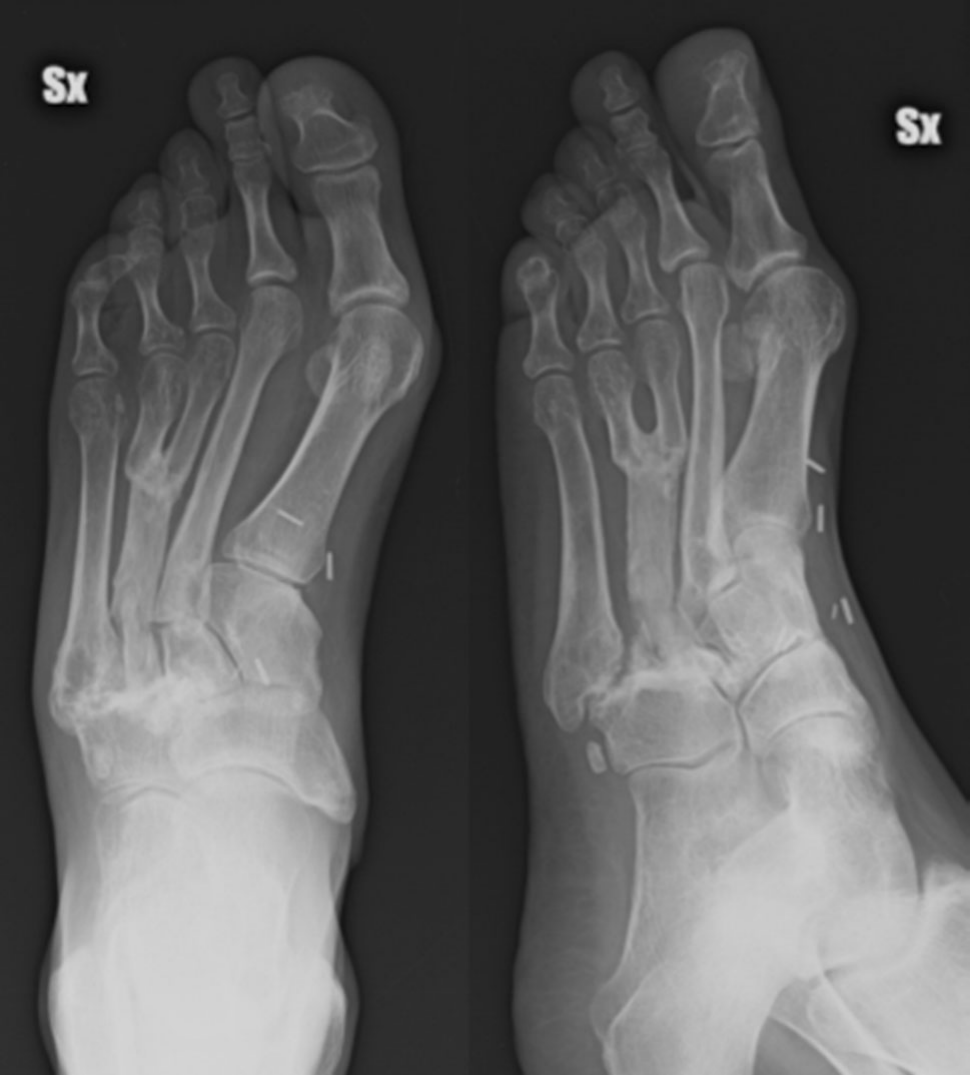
Radiographic oblique and antero-posterior view at follow-up
